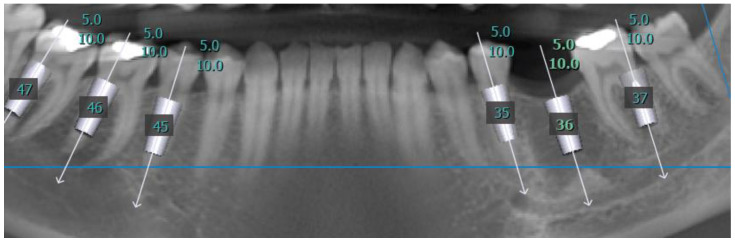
Virtual implant placement; positioning of 3 dental implants of 5 mm diameter and 10 mm long at each side of the mandibular sites: second premolar, distal root of first molar, distal root of second molar. CBCT panoramic reconstruction.
